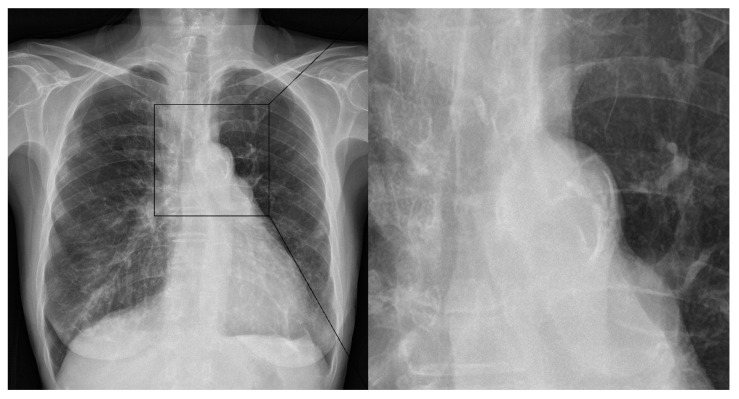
Assessment of aortic arch calcification using chest X-ray. Aortic arch calcification is assessed based on the presence of calcification.
